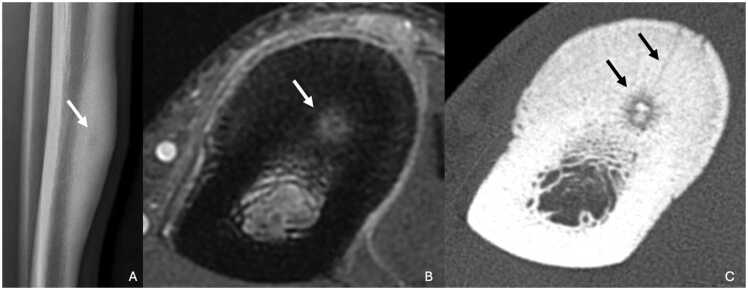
Osteoid osteoma of the diaphysis of the left tibia in a 16-year-old young man. A. Profile radiography showing thickening of the middle third of the tibial cortex with a central rounded clearness (white arrow). B. T1-weighted fat-suppressed contrast-enhanced MR image in axial plane showing a nodular enhancement of the anterior tibial cortex (white arrow) with periosteal enhancement. C. UHR CT in axial plane highlighting serpiginous low-density grooves radiating from periosteal surface to the central calcified nidus (medusa head sign, black arrows).
